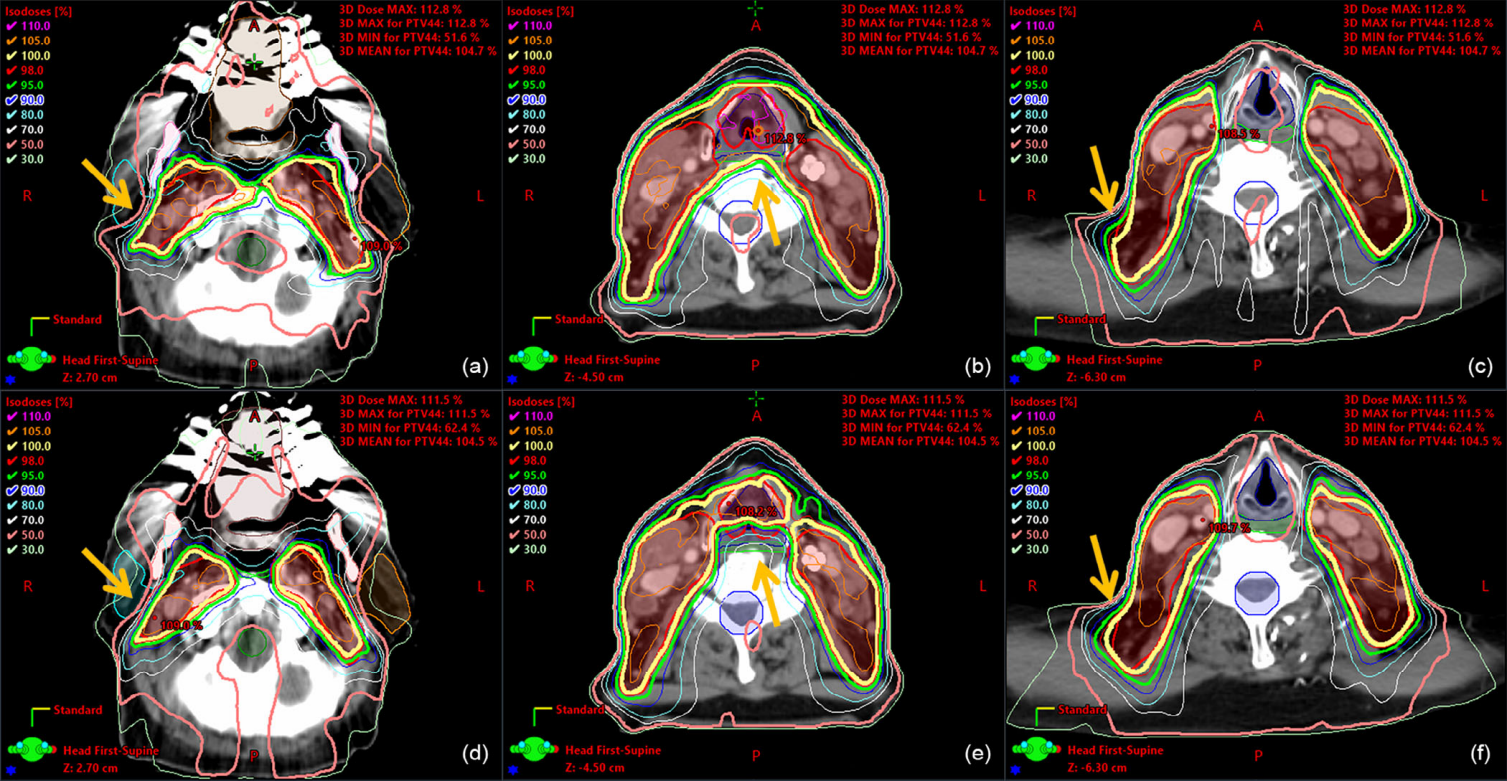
Dose distribution comparison of a commissioning case in axial view at different CT slices. (a–c) Clinical plan; (d, e) AI plan. Red: PTV44; Cyan: parotid right; Orange: parotid left; Green: brainstem; Blue: cord+5  mm; Dark blue: larynx; Green: pharynx; Brown: oral cavity. Yellow arrows indicate occasionally missed PTV coverage in both plans.
